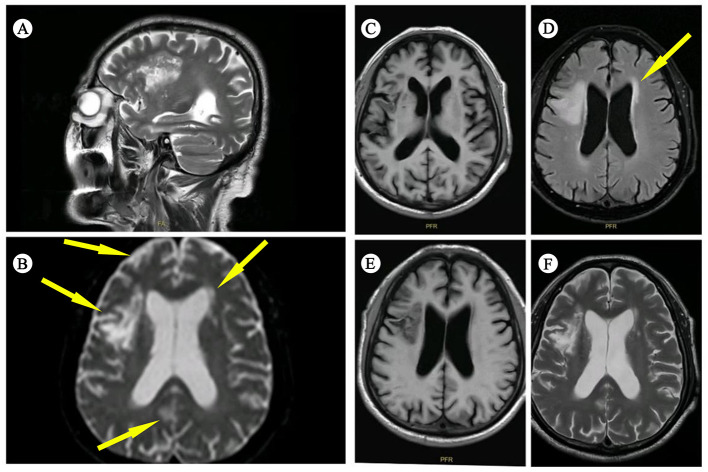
Brain magnetic resonance imaging findings. (A) Sagittal T2-weighted fast spin echo sequence. (B) Axial diffusion-weighted imaging at the ventricular level. (C) Axial T1-weighted fluid-attenuated inversion recovery sequences. (D) Axial T2-weighted fluid-attenuated inversion recovery sequence with fat suppression. (E) Axial T1-weighted fluid-attenuated inversion recovery sequences. (F) Axial T2-weighted fast spin echo sequence.
